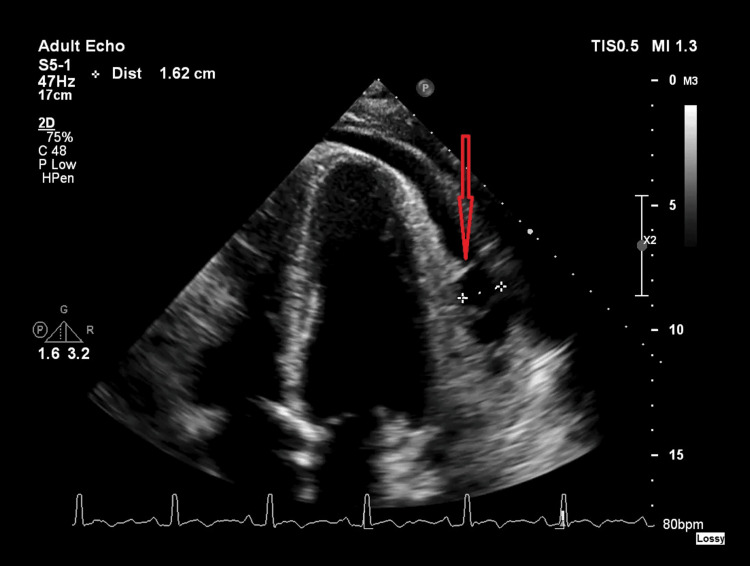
Echocardiography apical view. The figure reveals the pericardial effusion with a septa posterior to the left ventricle.
